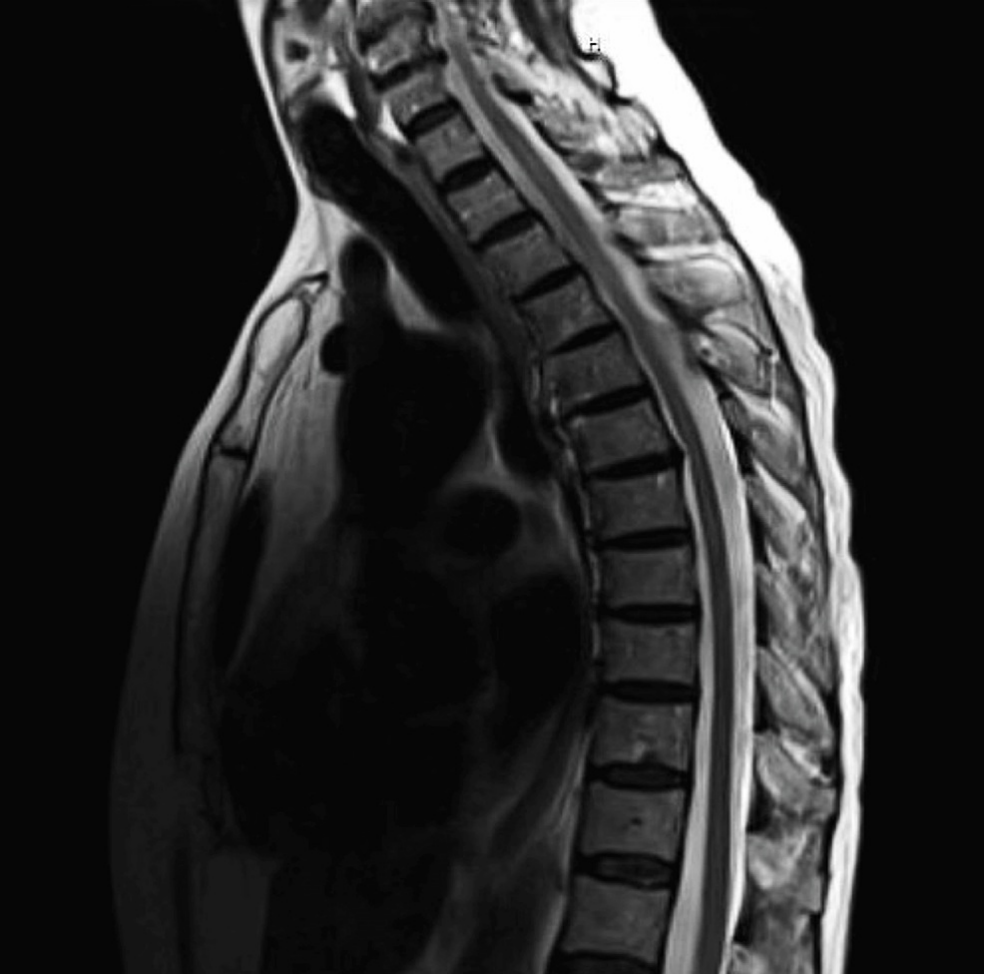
Repeat T2-weighted thoracic spine MRI on day 8, sagittal view, showing decreased contrast enhancement throughout the thoracic spine MRI: magnetic resonance imaging
